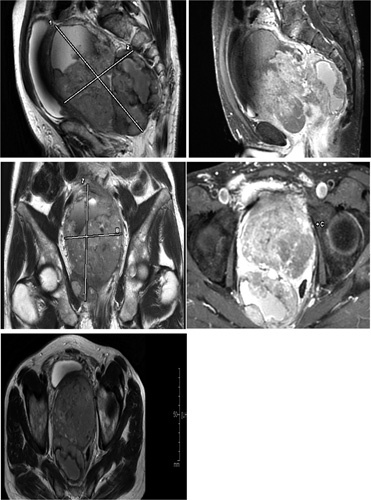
Magnetic resonance image of the prostate (our case).
